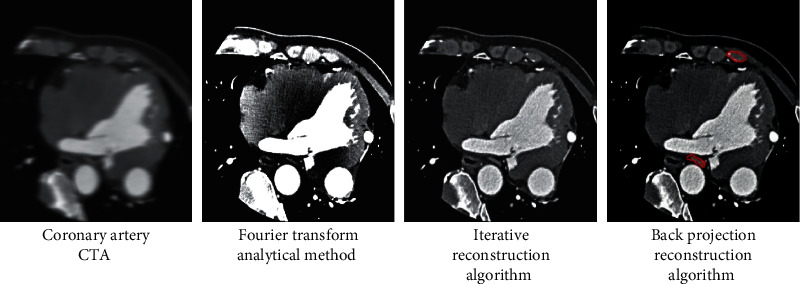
Coronary CTA image reconstruction. (a) Coronary CTA image; (b) Fourier transform analysis
